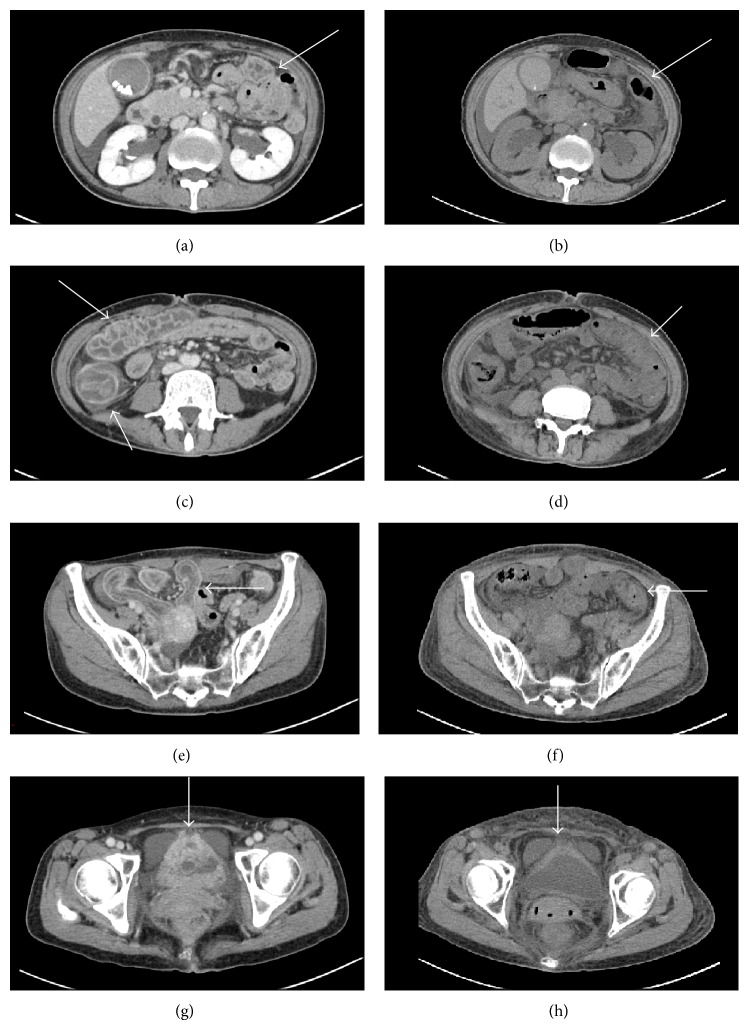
Enhanced abdominal CT on admission revealed an enlarged gall bladder (a, arrow), hydronephrosis, and ascites. The wall thickening with a marked three-wall structure extended from the terminal ileum to the sigmoid colon (c, e, arrows), and the bladder wall was irregularly thickened and enhanced (g, arrow). Eight days later, plain abdominal CT revealed an almost normal gall bladder wall (b, arrow), alimentary tract (d, f, arrow), and bladder (h, arrow).
